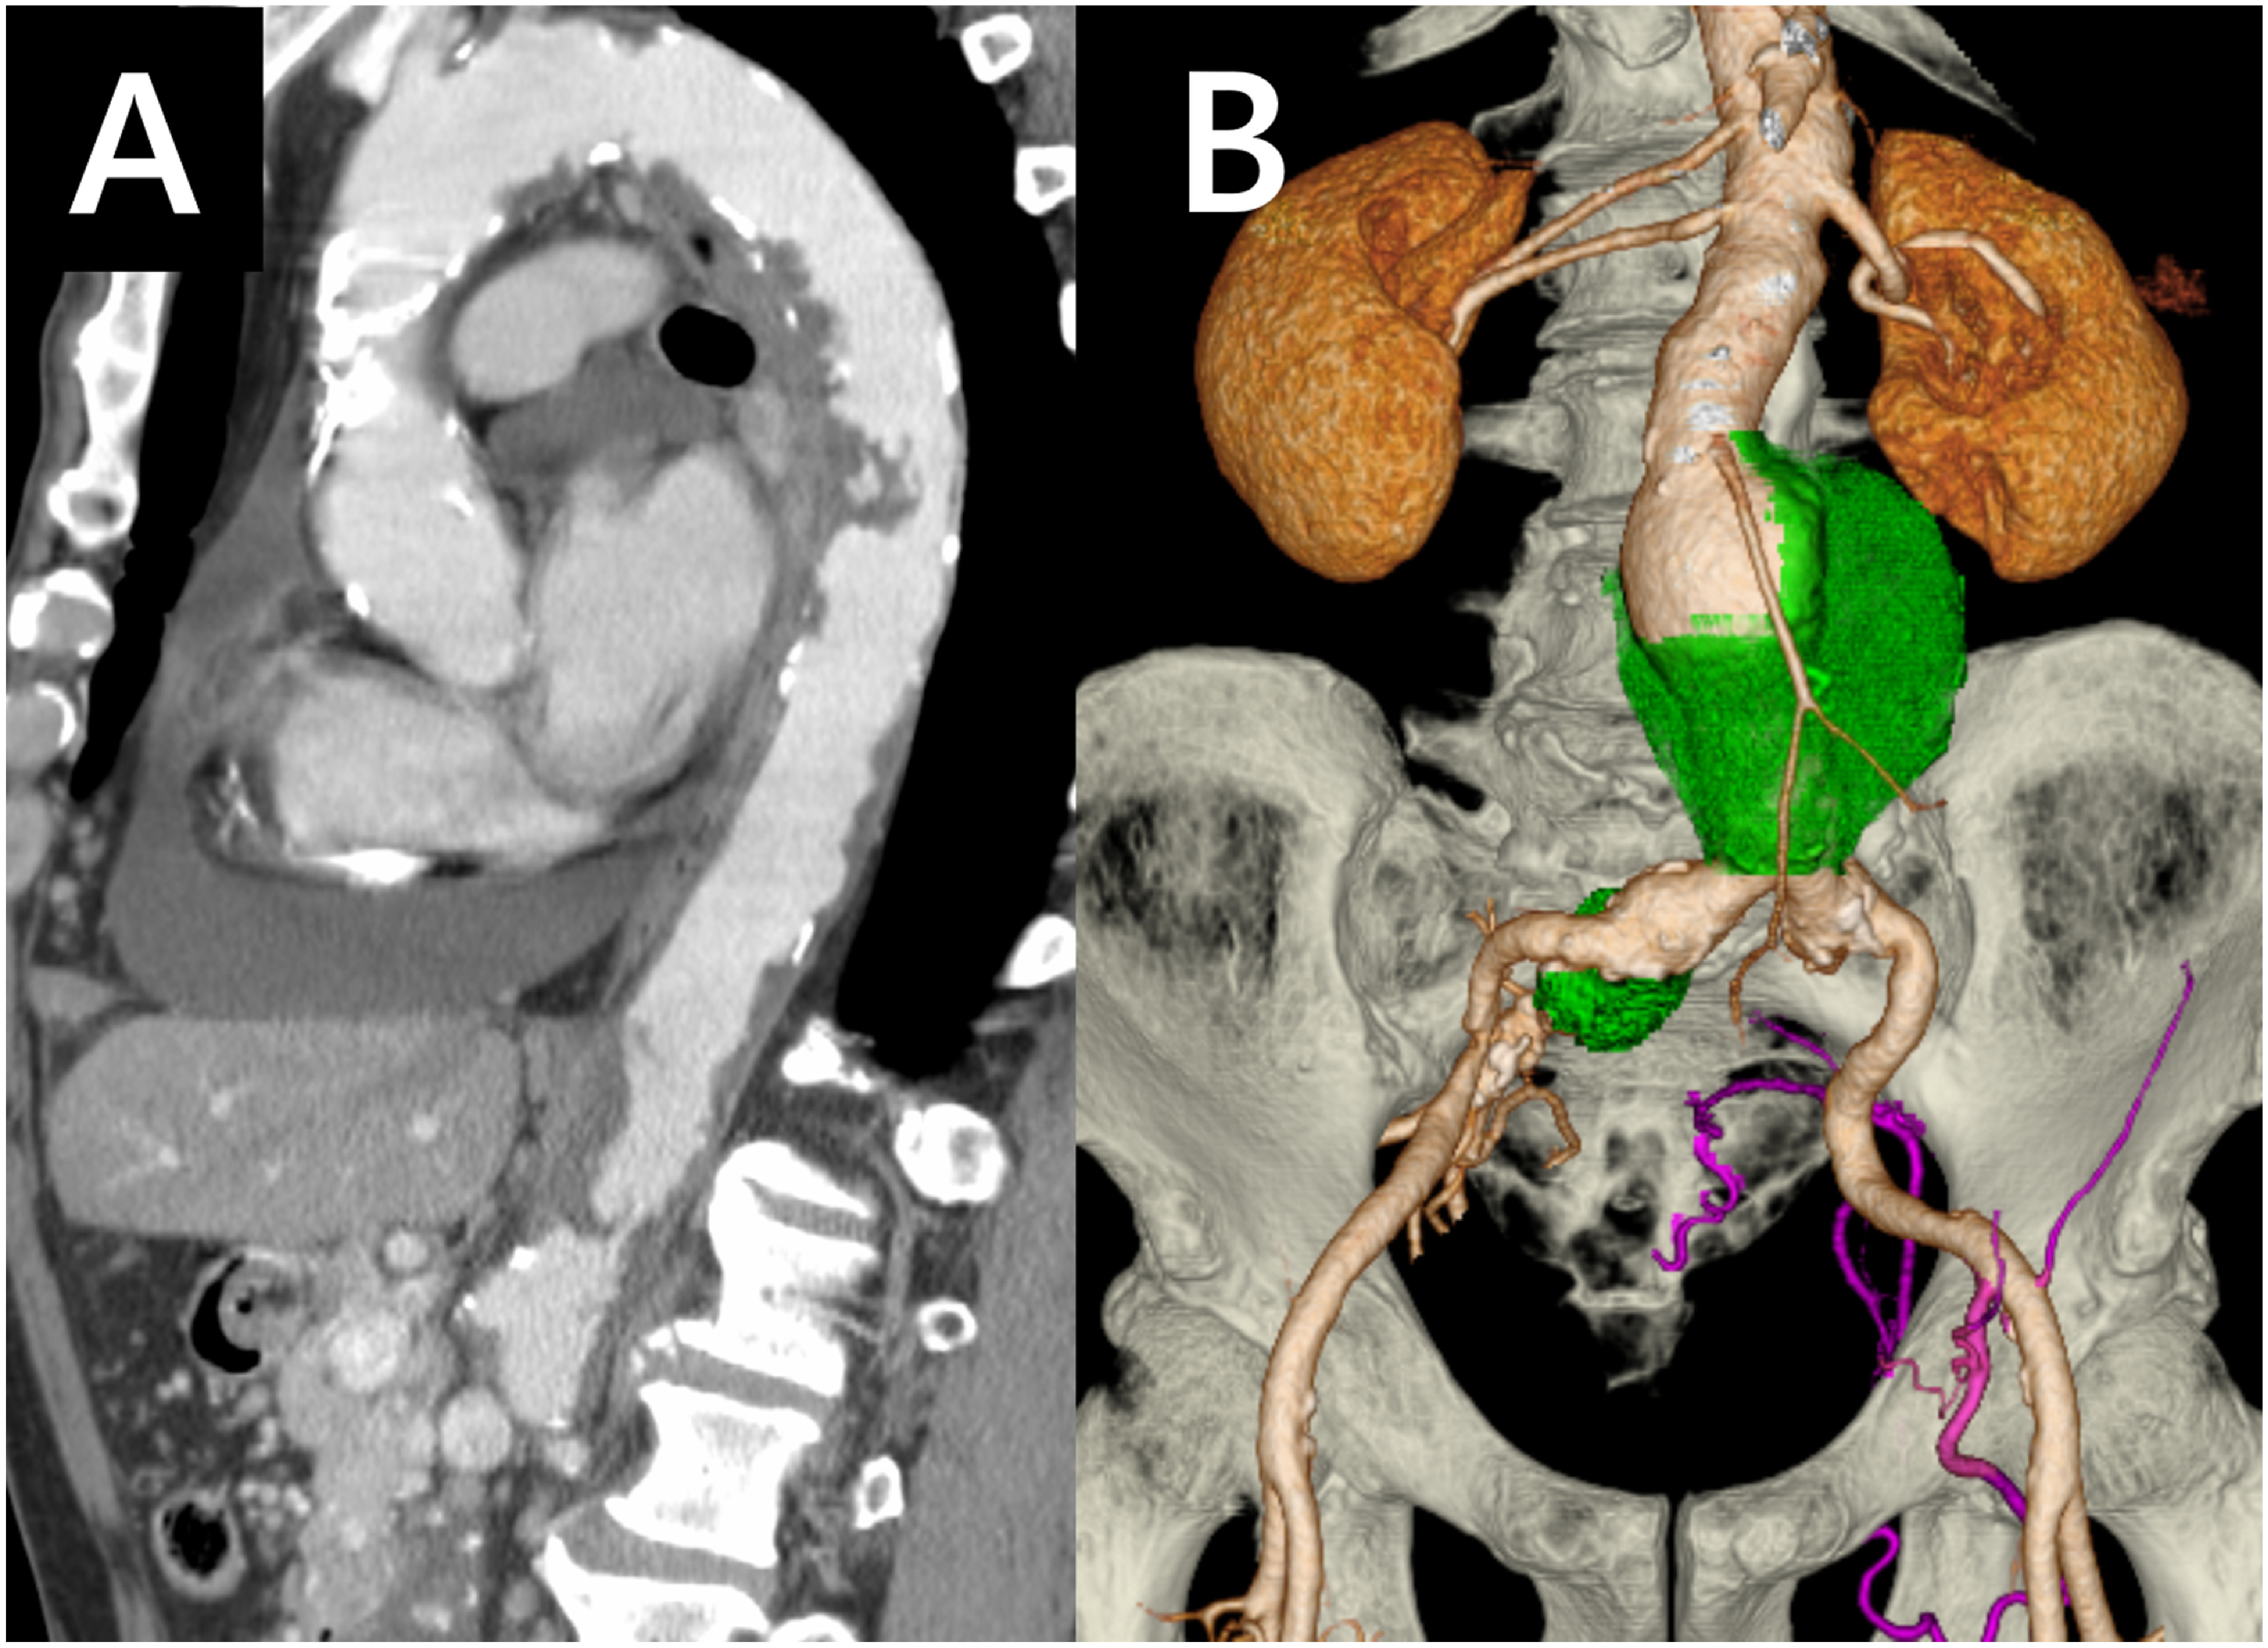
Fig. 2 (A) Contrast-enhanced computed tomography images conducted 4 years before the current presentation. The aorta has very severe atherosclerotic changes and mobile plaques. (B) Preoperative three-dimensional computed tomography in Case 3 shows a 56 mm infrarenal abdominal aortic aneurysm, a 26 mm right internal iliac artery (IIA) aneurysm, and collateral supply from the left femoral artery to the distal end of the occluded left IIA (pink color).
